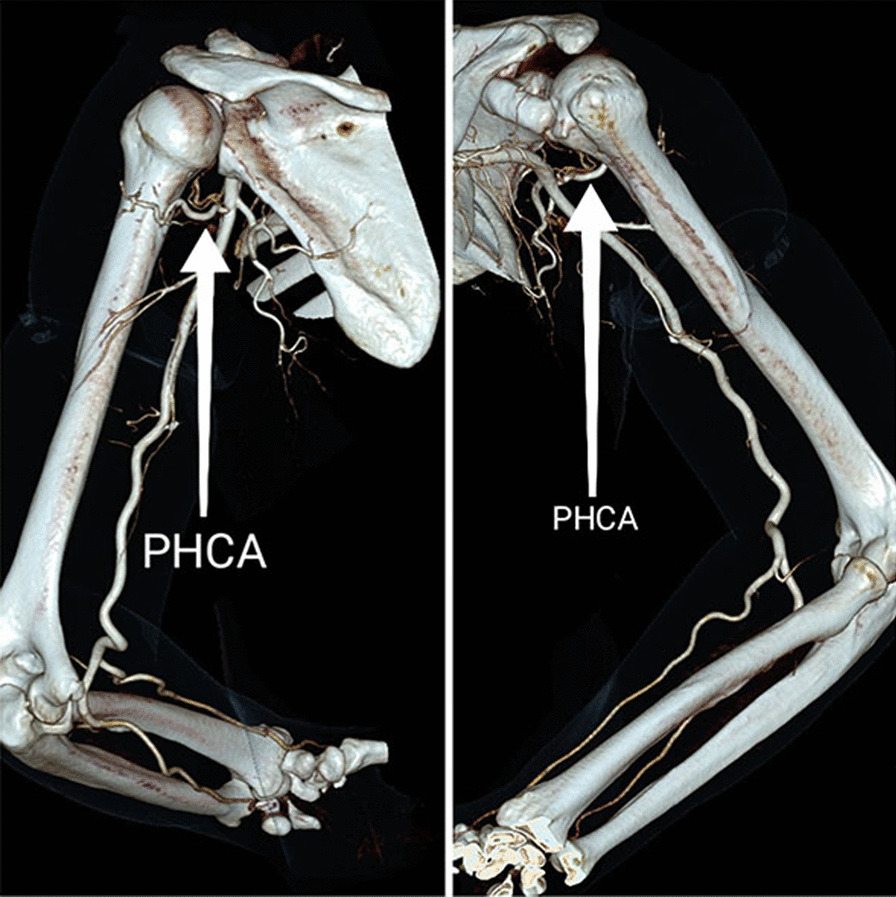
CTA of upper left limb. The ACHA is difficult to visualize in CTA
